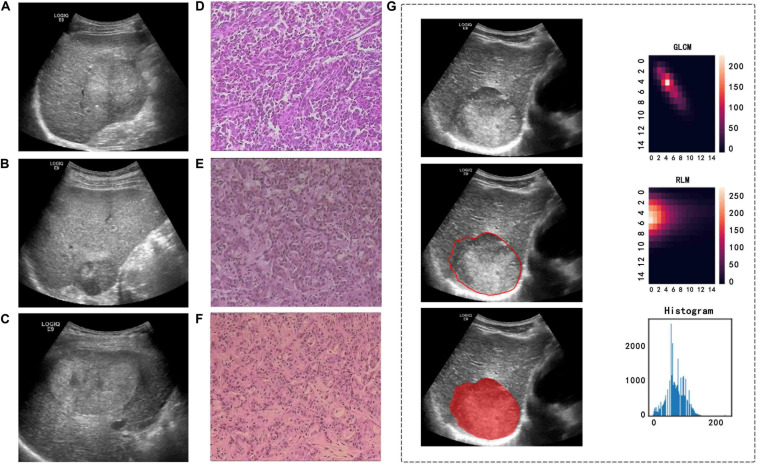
Ultrasound and pathological images, tumor segmentation, and feature extraction of three different pathological types of PLC. (A,D) A 57-year-old man with a pathological diagnosis of cHCC-ICC. (B,E) A 64-year-old woman with a pathological diagnosis of HCC. (C,F) A 44-year-old man with a pathological diagnosis of ICC. (G) An example of manually sketching the region of interest (ROI) of a tumor on an ultrasound image and gray level co-occurrence matrix (GLCM) features, run length matrix (RLM) features, and grayscale histogram feature extraction from the grayscale ultrasound image.
